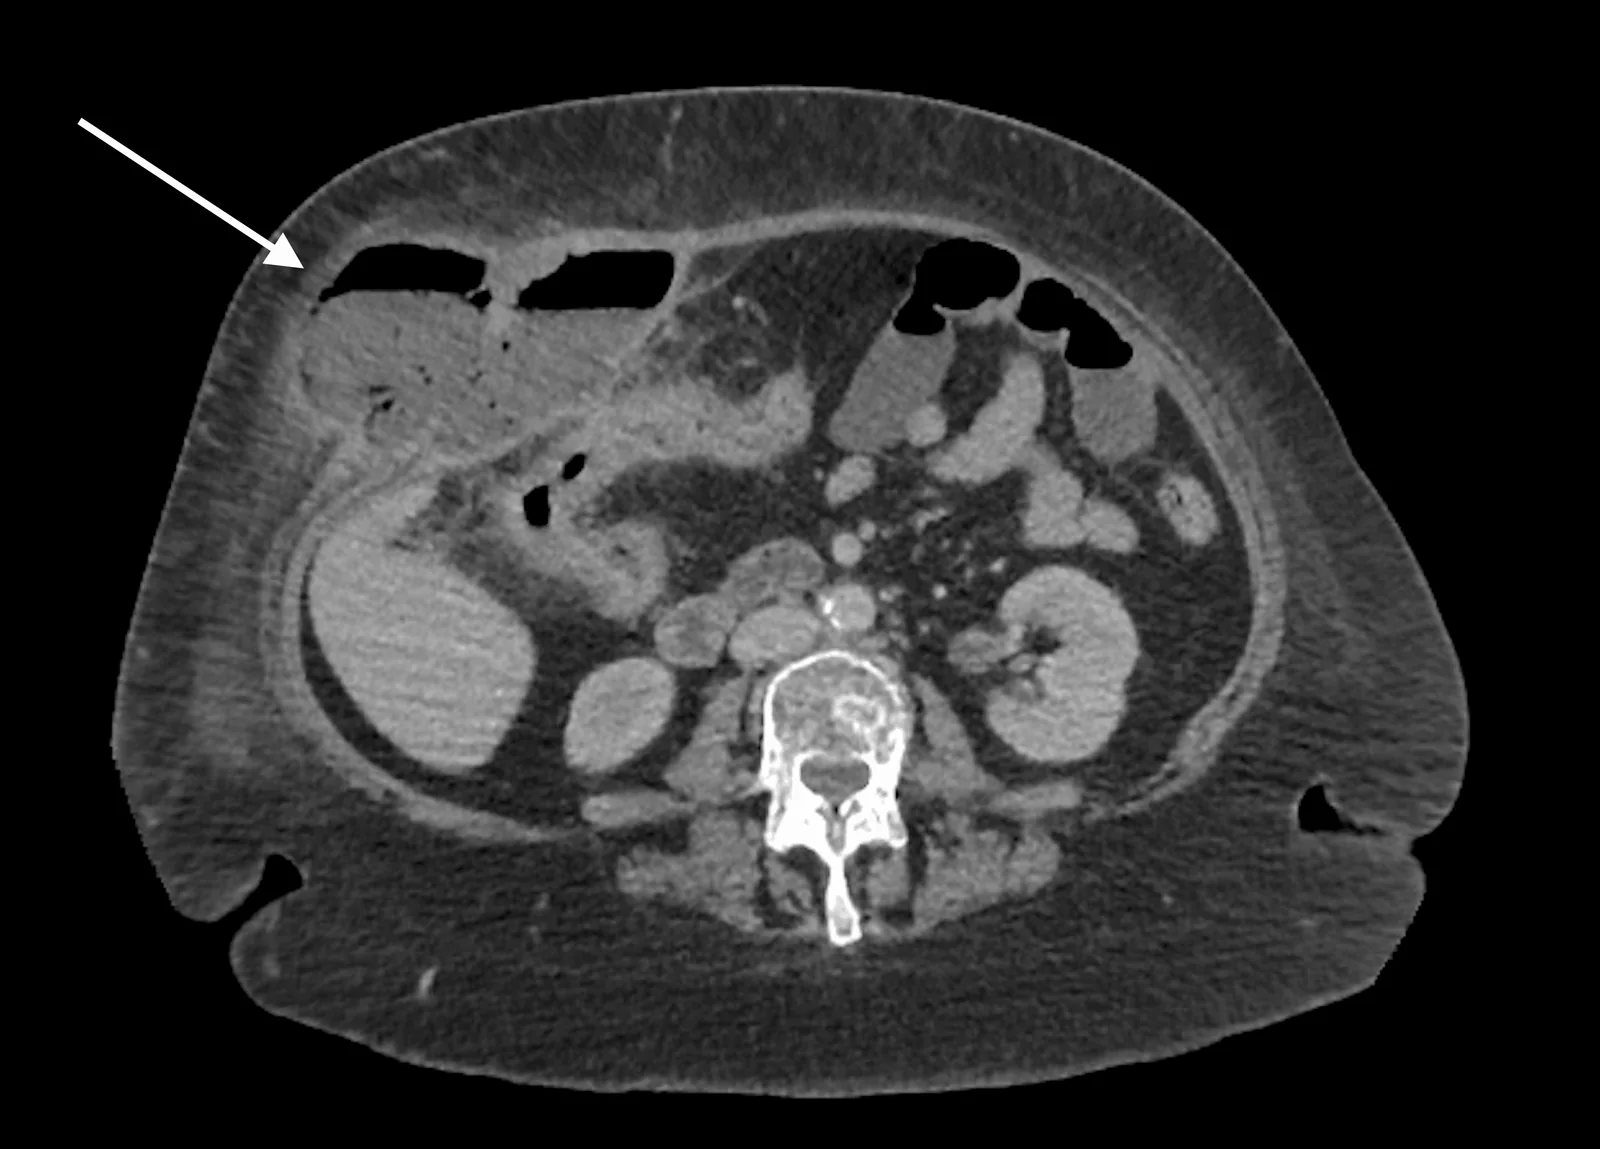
CT scan abdomen and pelvis with intravenous contrast, with arrow pointing to acute cutaneous abscess portion of fistula
